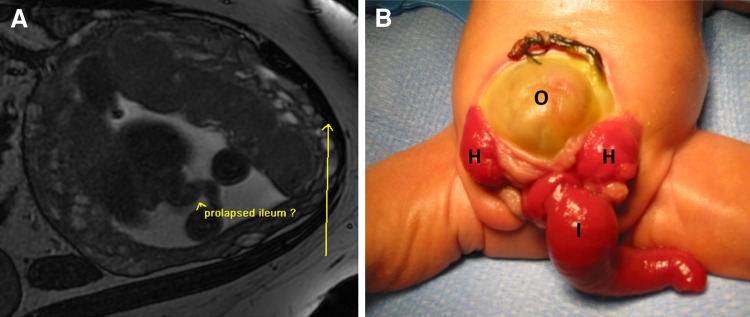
Patient with cloacal exstrophy showing the elephant trunk sign representing the intussuscepted ileum. a Prenatal MRI. b Postnatal image (*o* omphalocele, *h* hemibladders, *i* “elephant trunk” ileum)
